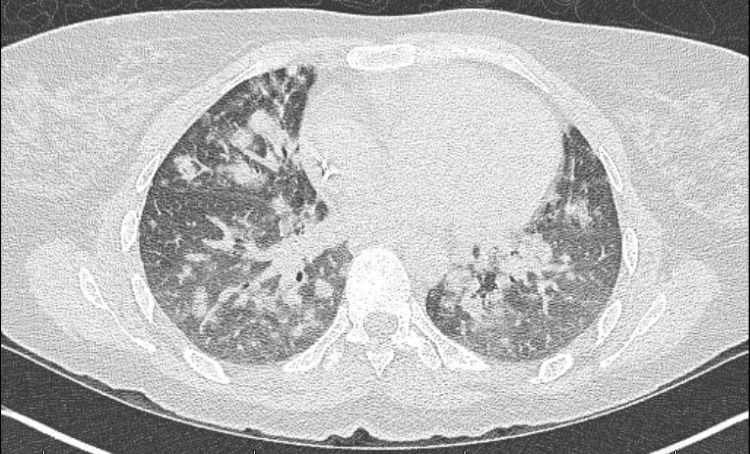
The patients high-resolution chest computed tomography scan showing mid and lower lobe predominant peribronchovascular ground-glass and consolidative nodular opacities.
